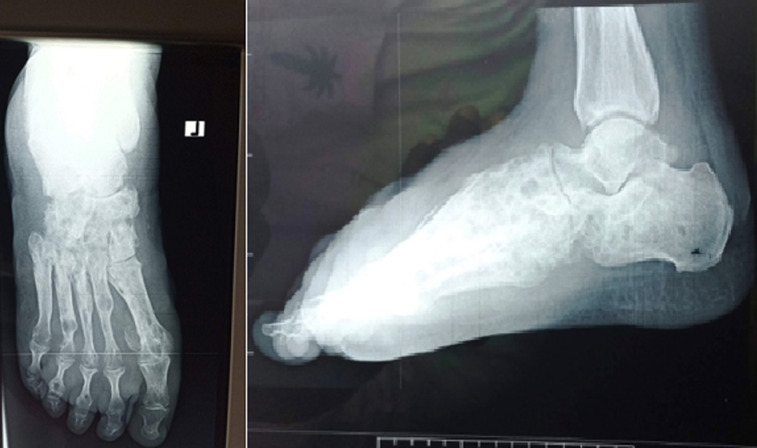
anterior-posterior x-ray of the left foot showing non-specific bone destruction
